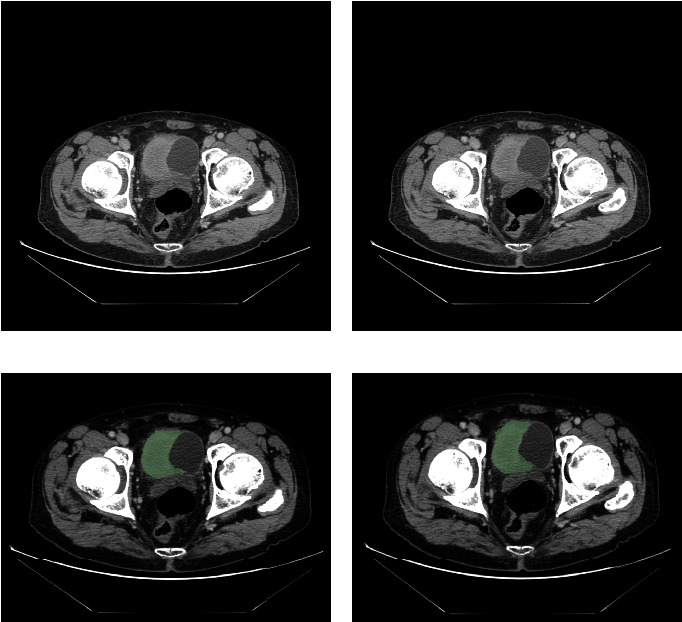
(a, b) The CT image of a 65-year-old bladder cancer patient and (c, d) the ROI of the CT image of a 65-year-old bladder cancer patient.
